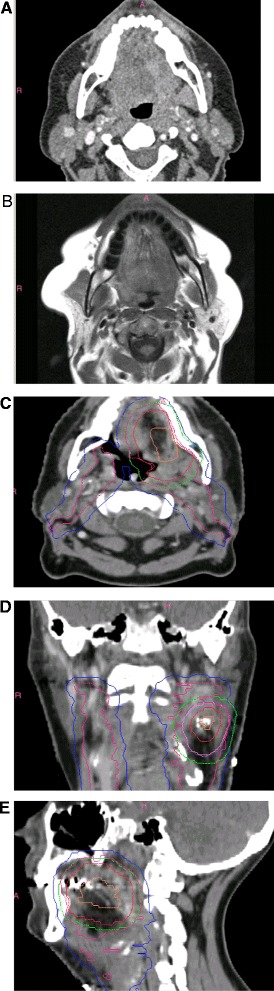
Dummy run case imaging. Representative preoperative (A) computed tomography and (B) axial T2-weighted magnetic resonance image provided. (C) Axial, (D) coronal and (E) sagittal display of master contours. Orange: GTV-pt. Magenta: CTV52.8 Gy. Pink: CTV59.4 Gy. Red: CTV66 Gy. Blue: PTV52.8 Gy-Exact*. Green: PTV2Exact*. Purple: PTV3Exact*. *PTVExacts denote PTVs collapsed inside the skin by 5 mm.
